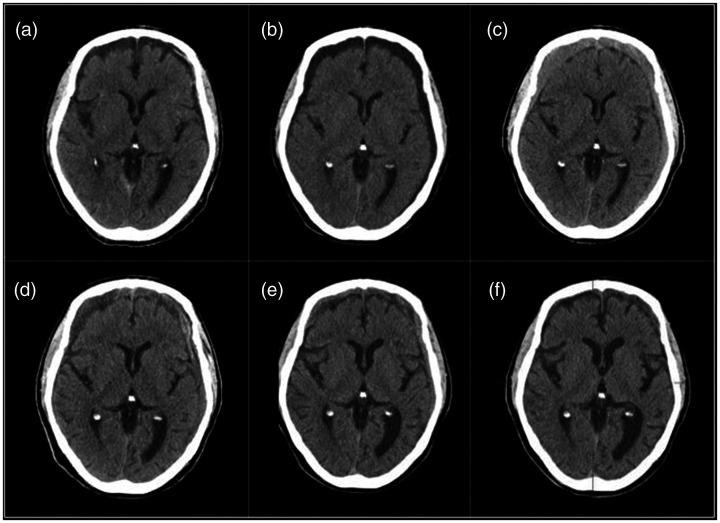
Forty-seven year old (a) initially with a small SDH on CT, (b) showing slight enlargement after three weeks. (c) Acute blood developed once anticoagulation was restarted. MMA embolization was performed and imaging at (d) 6 weeks, (e) 5 months, and (f) 12 months post-embolization showed resolution.
